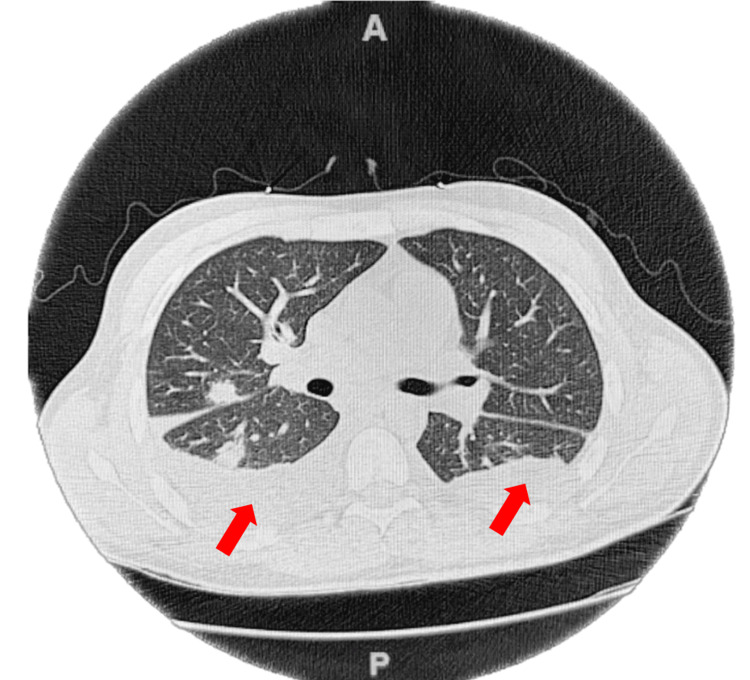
Computed tomography (CT) of the chest in axial view showing bilateral pleural effusions (red arrows), and a moderate right lower lobe consolidation concerning for pneumonia.
